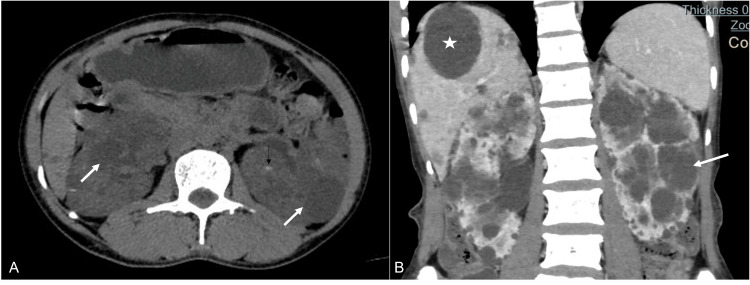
A young male patient with recurrent lumbar pain Axial NCCT (A) and coronal reformatted CECT (B) images show multiple hypodense cysts randomly distributed in both kidneys (short white arrows), which are classified as Bosniak I cysts. A few cysts show internal hyperdense fluid layering, suggesting internal haemorrhages (black arrow), which are categorized as Bosniak II cysts. The liver also shows multiple simple cysts (white asterisk). NCCT: non-contrast-enhanced CT; CECT: contrast-enhanced CT
